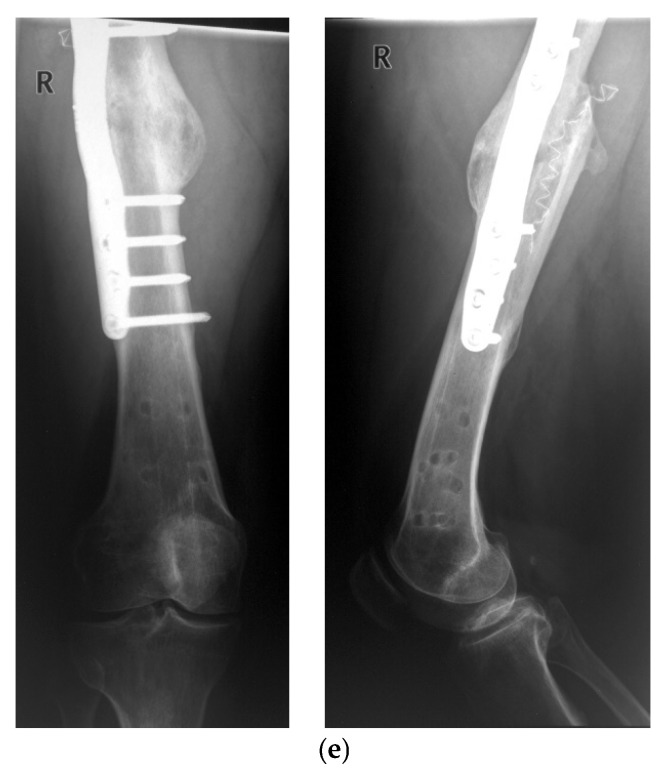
Anteroposterior radiographs preoperatively with a nail inserted (a), 5 days postoperatively (b), 8 weeks postoperatively (c) and 16/20 weeks postoperatively (d,e) are shown.
